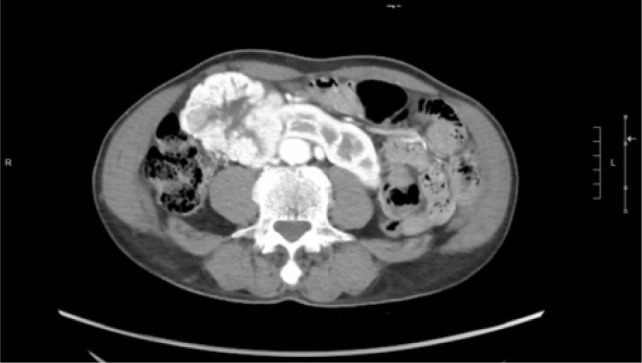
Axial cut of arterial phase-enhanced CT abdomen at the tumor level.
